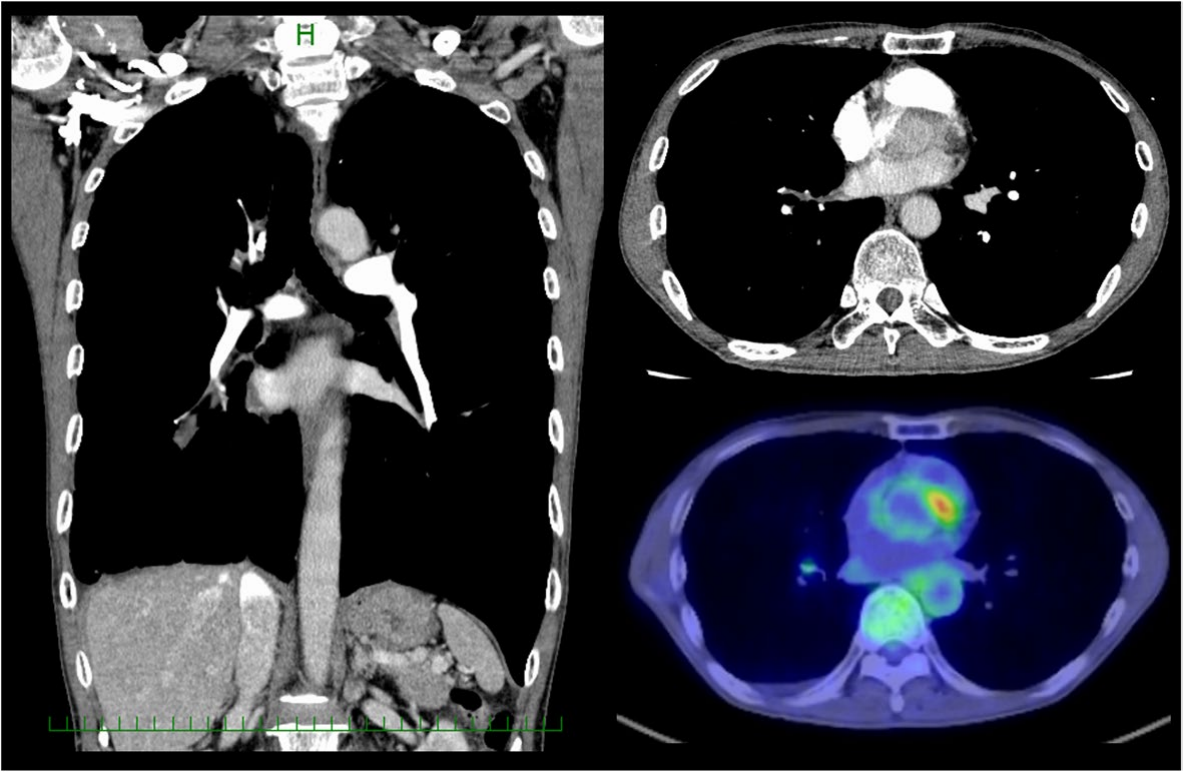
Computed tomography and positron emission tomography after osimertinib treatment. Computed tomography after 4 months’ osimertinib treatment (coronal section [left] and transverse section [right upper]) showed a marked tumor shrinkage. No apparent tumor invasion into the left antrum was observed. Positron emission tomography revealed no significant glucose uptake with the maximum standardized uptake value of 1.7 (right lower)
